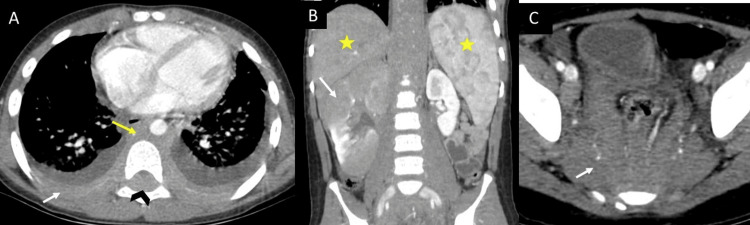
(A) Contrast-enhanced chest CT scan in axial section showing infiltrative involvement of the paravertebral mediastinum (yellow arrow) extending into both pleural cavities with pleural effusion and thickening (white arrow), and extending into the epidural space of the spinal canal (black arrowhead). Contrast-enhanced abdomen and pelvis CT scan in coronal (B) and axial (C) sections showing hepatosplenomegaly (yellow stars in B), right nephromegaly with multiple ill-defined hypodense lesions in the kidney and perirenal space (white arrow in B). Infiltrative-looking mass in the retroperitoneum, presacral space, and pelvic side walls (white arrow in C).
